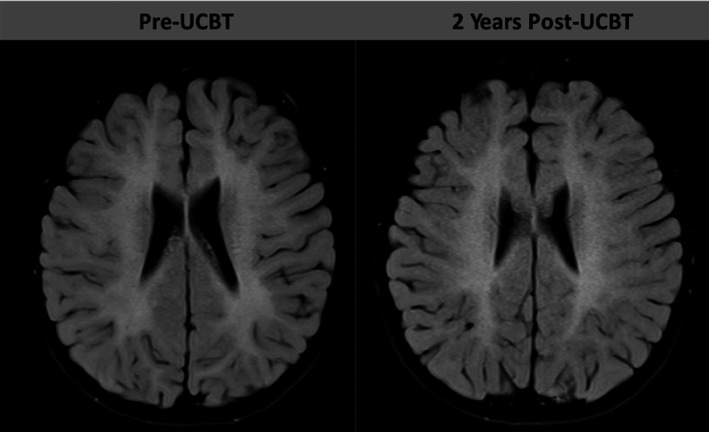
Representative axial FLAIR sequences from brain MRI. Left MRI was performed at age 4 years, 8 months (pre‐UCBT) and at age 6 years, 8 months (2 years post‐UCBT) in a boy with β‐mannosidosis
